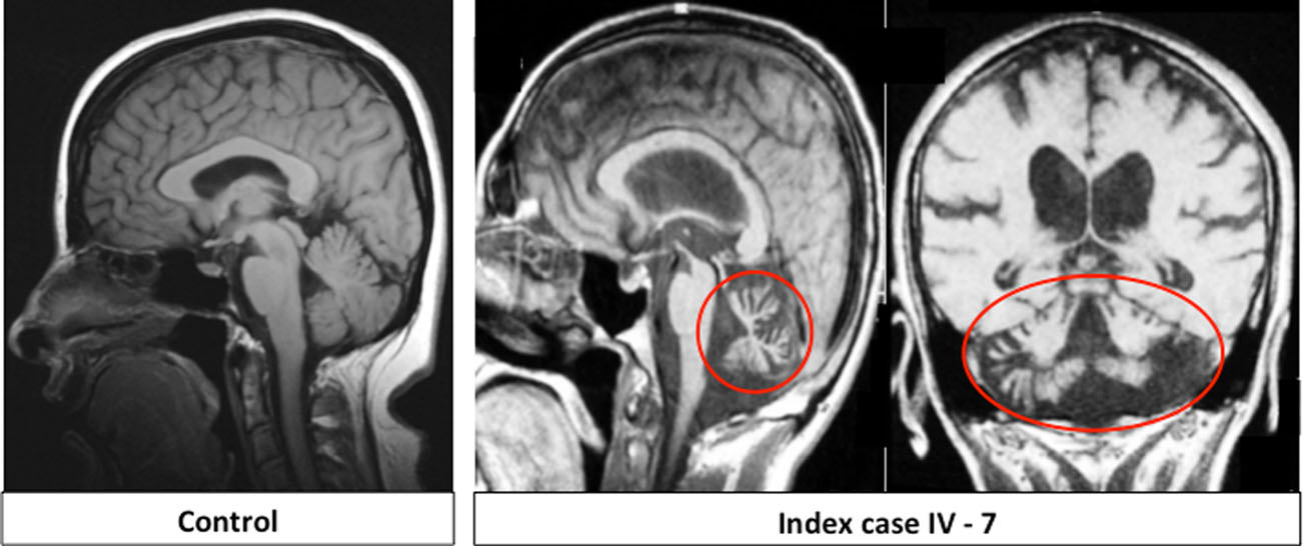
T1-weighted sagittal MRI of unaffected control proband (*left*) and sagittal and coronal MRI of index case at age 60 depicting severe cerebellar atrophy circled in *red* and some additional degree of midbrain atrophy
